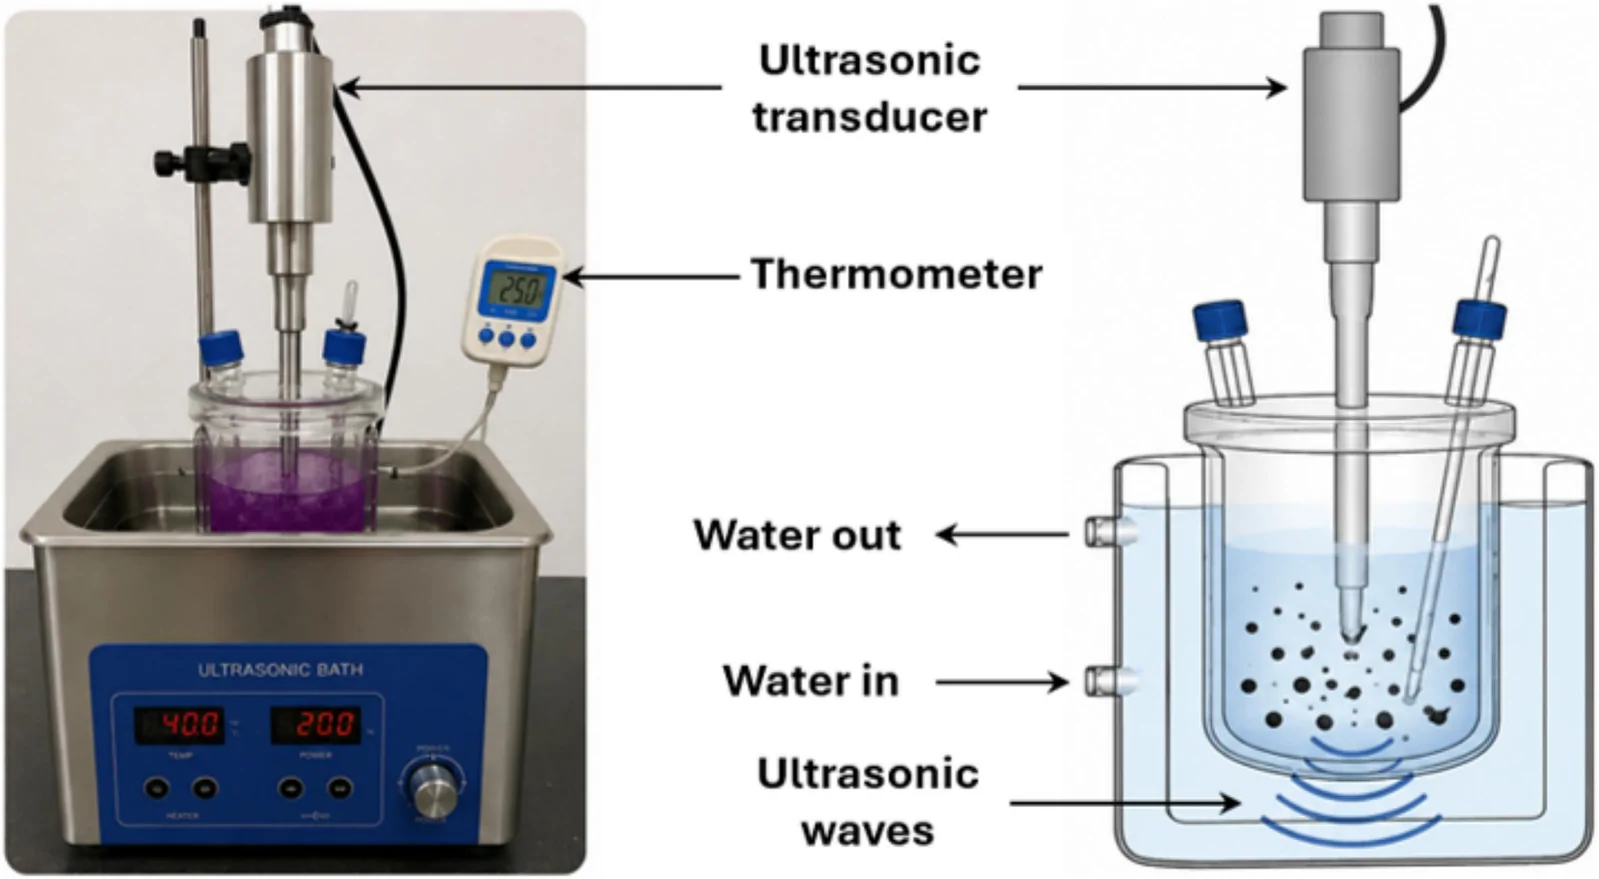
Ultrasound-assisted batch adsorption reactor.
